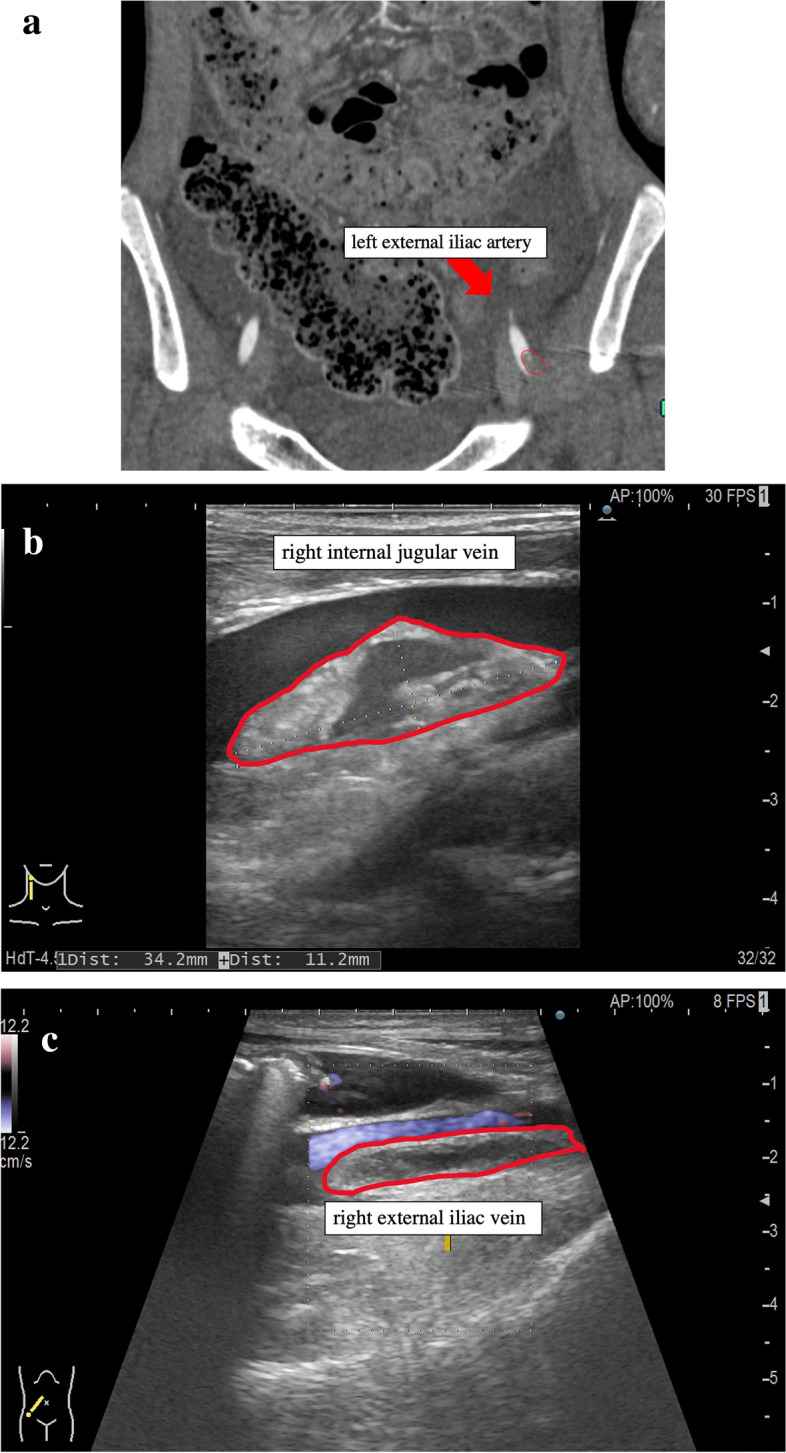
Detection of multiple thrombi by contrast-enhanced computed tomography (CE-CT) and venous ultrasonography. a Sagittal view of CE-CT. A thrombus is seen in the wall of the left external iliac artery (inside the red circle). b Venous ultrasonography of the right internal jugular vein. A thrombus is seen in the wall of the right internal jugular vein (inside the red circle). c Venous ultrasonography of the right external iliac vein. A thrombus is seen in the wall of the right external iliac vein (inside the red circle)
